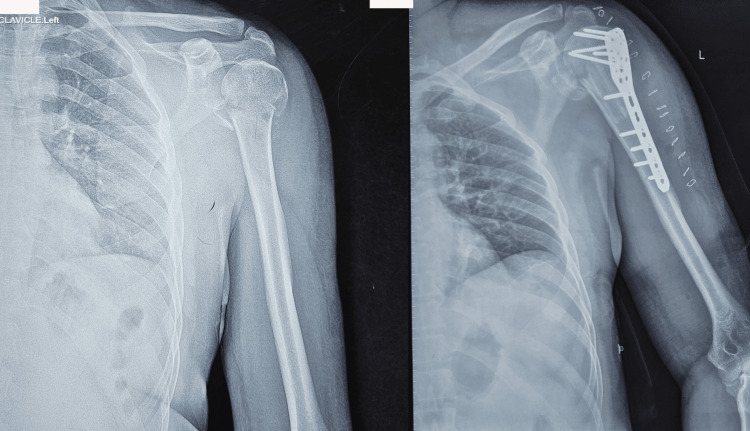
Preoperative radiograph of the shoulder demonstrating a displaced proximal humerus fracture and Immediate postoperative radiograph showing PHILOS plate fixation augmented with an autologous intramedullary fibular strut graft PHILOS: Proximal humerus internal locking system
